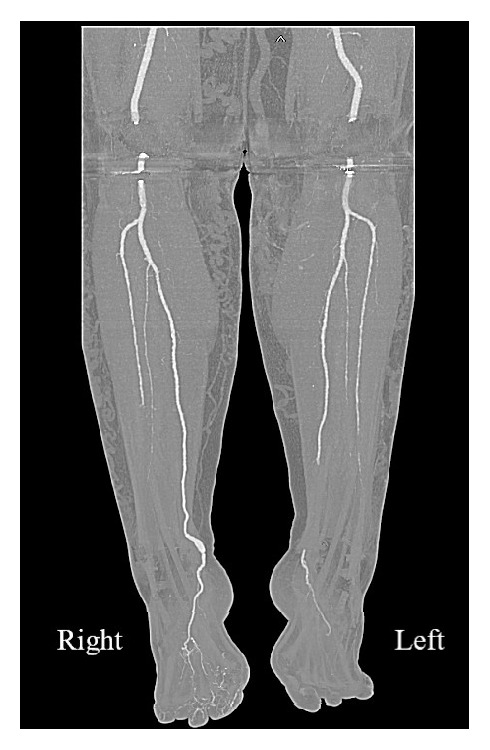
Preoperative reconstructed computed tomography angiography of the tibial arteries bilaterally, demonstrating abrupt occlusion of the left posterior tibial artery and a right-sided posterior tibial artery aneurysm. Please note that artefact from bilateral knee prostheses obstructs visualising normal flow within the popliteal arteries in this image.
